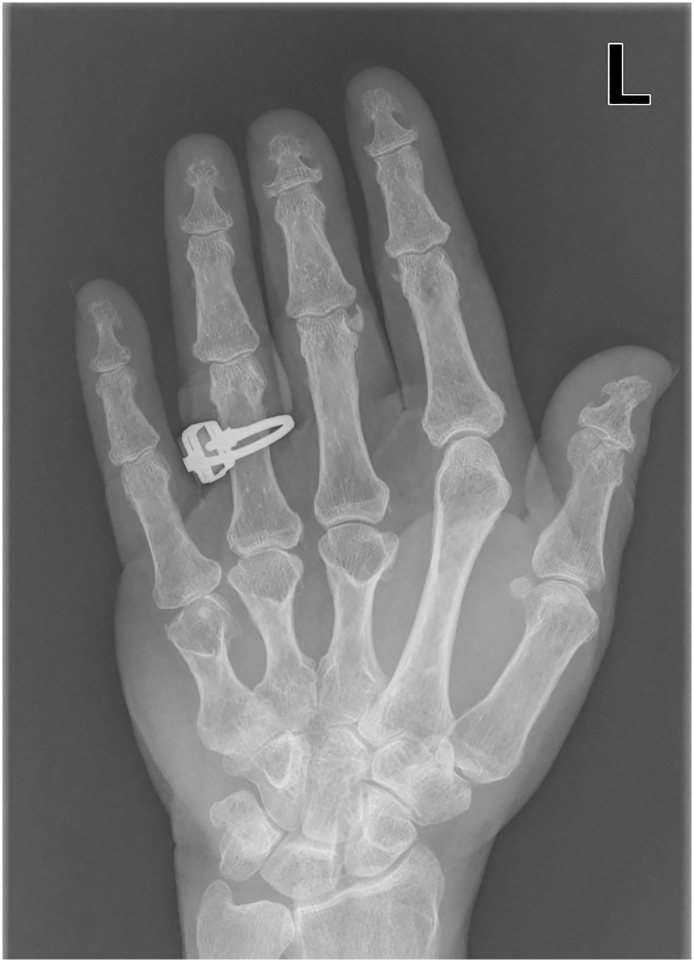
Radiography of the left hand with evident brachydactyly of metacarpals III-V.
